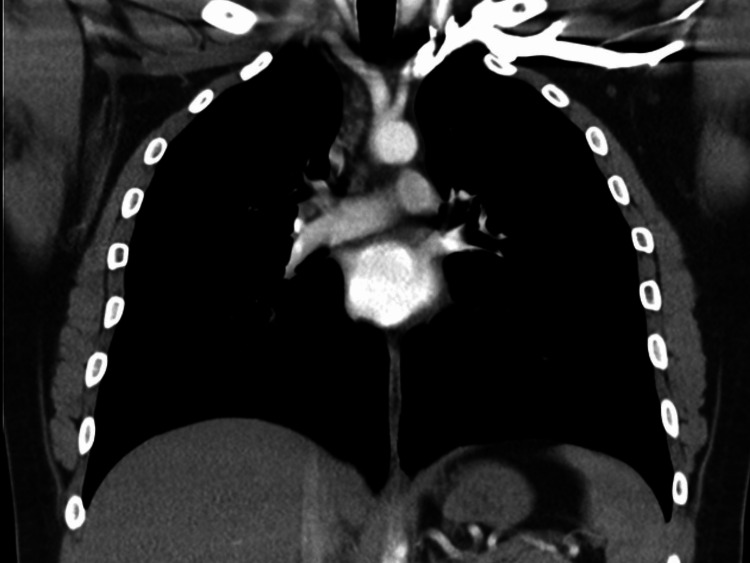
Normal CT image of the chest
